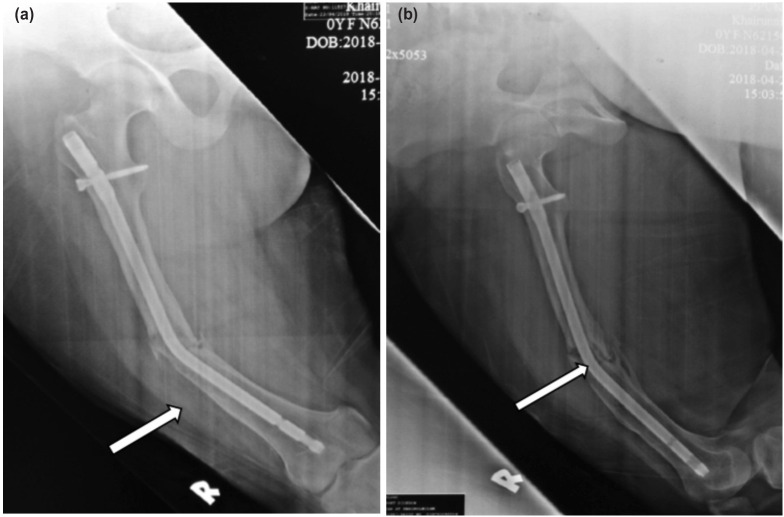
(a) Plain radiograph shown 30° varus angulation of femoral nail in AP view (b) and 30° anterior angulations in lateral view with the continuity of the nail was intact. It also shown that the femoral shaft fracture was not united with unicortical callus formation.
